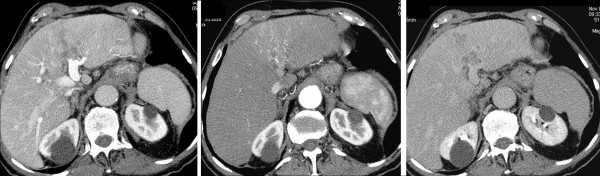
Baseline CT examination: 1a, arterious phase; 1b, portal phase; 1c, late phase. Between III and IV hepatic segment is possible to observe the presence of a solid lesion with poorly defined margins and infiltrative nature; the lesion is hyperdense in arterial phase and hypodense in the portal phase and late, and it invades the left main portal vein which is thrombosed.
